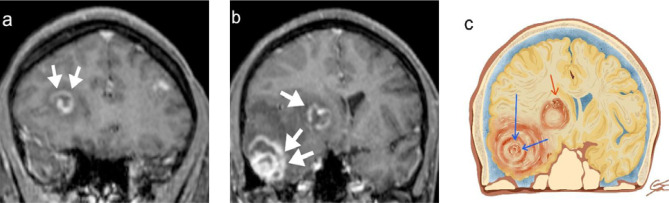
MRI of a 37-year-old male AIDS patient with left hemiparesis.
